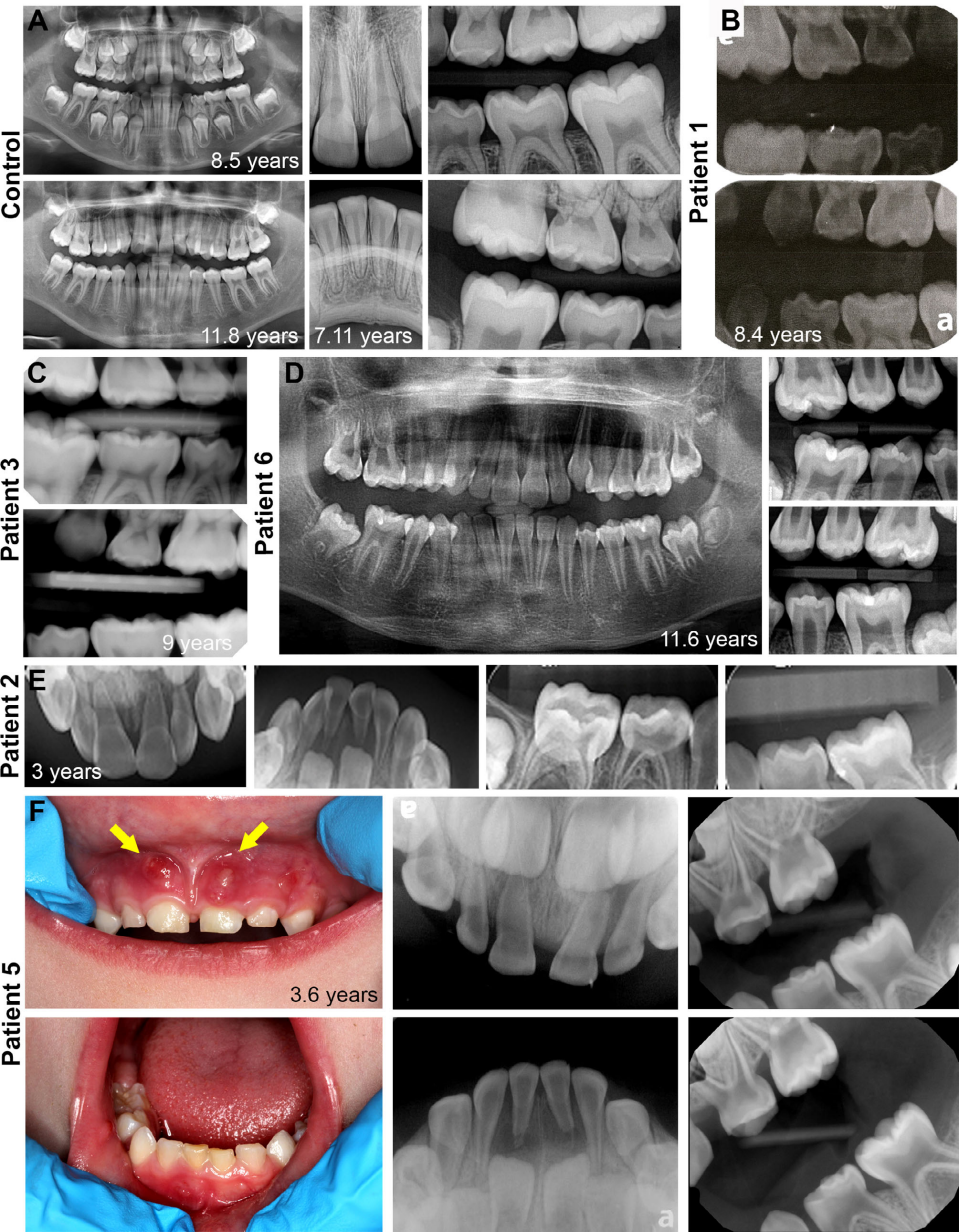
Clinical and radiographic effects of X‐linked hypophosphatemia rickets (XLH) on the dentition. (*A*) Dental panoramic, periapical, and bitewing radiographs from a representative healthy control individual at ages of 7 years 11 months, eight years five months, and 11 years 8 months. (*B*) Bitewing radiographs from XLH patient 1 aged 8 years 4 months showing normal enamel, dentin, and pulp chamber appearance. (*C*) Bitewing radiographs from patient 3 at 9 years of age showing normal enamel, dentin, and pulp chamber appearance. (*D*) Panoramic and bitewing radiographs from patient 6 aged 11 years 6 months shows relatively normal pulp chambers and canals in the adult dentition. (*E*) Periapical and bitewing radiographs from patient 2 aged 3 years indicate thin enamel, thin dentin, and enlarged pulp chambers in anterior teeth. (*F*) Oral photographs from patient 5 aged 3 years 6 months show the presence of fistulas (yellow arrows) near maxillary incisors. Periapical and bitewing radiographs show thin enamel, thin dentin, and enlarged pulp chambers.
